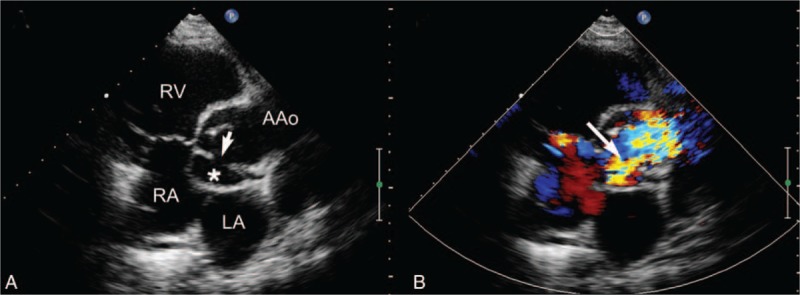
Real-time 3-dimensional transthoracic echocardiography showing P-MAIVF. The pocket-like lesion in the region of mitral aortic intervalvular fibrosa protrudes into the LA in the left ventricle long-axis view. Real-time 3-dimensional transthoracic echocardiography shows the oval orifice between the left ventricular outflow tract and P-MAIVF in the left ventricular view (arrow). MV = mitral valve; P-MAIVF = pseudoaneurysm of the mitral-aortic intervalvular fibrosa.
